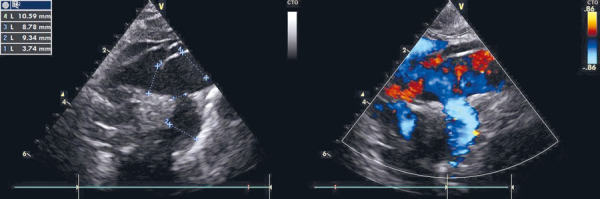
Echocardiography after birth showing a dilated a. subclavia sinistra
